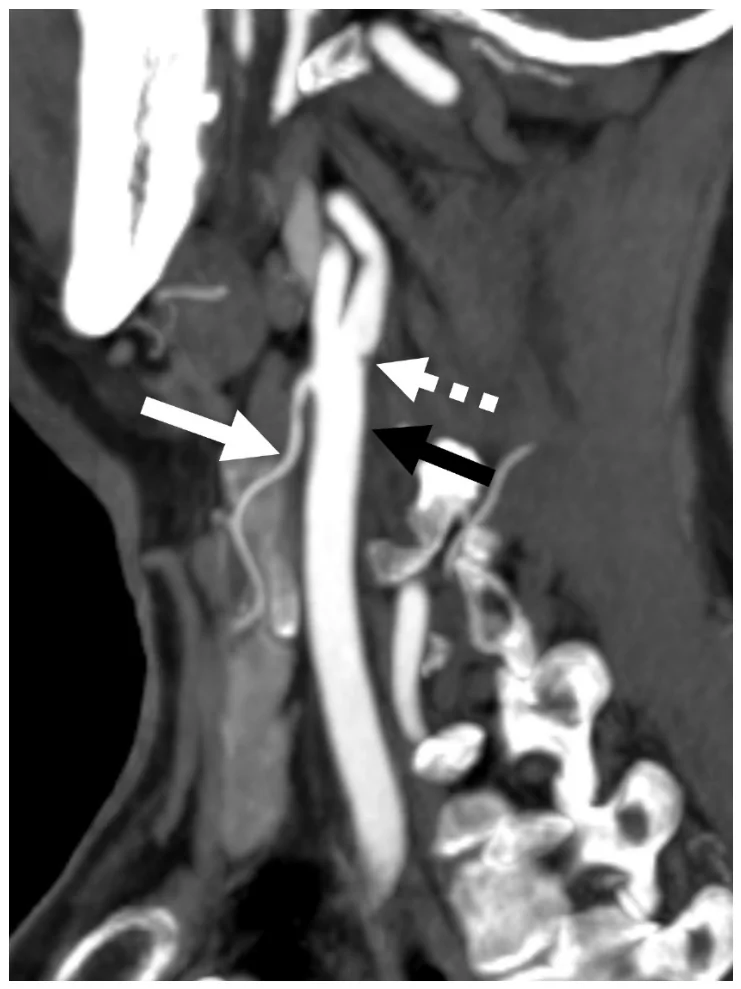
Superior thyroid artery originating from the CCA. Sagittal MIP CTA image shows the left superior thyroid artery (white arrow) arising from the CCA (black arrow) just proximal to the carotid bifurcation. An incidental carotid web is noted (dashed white arrow).
